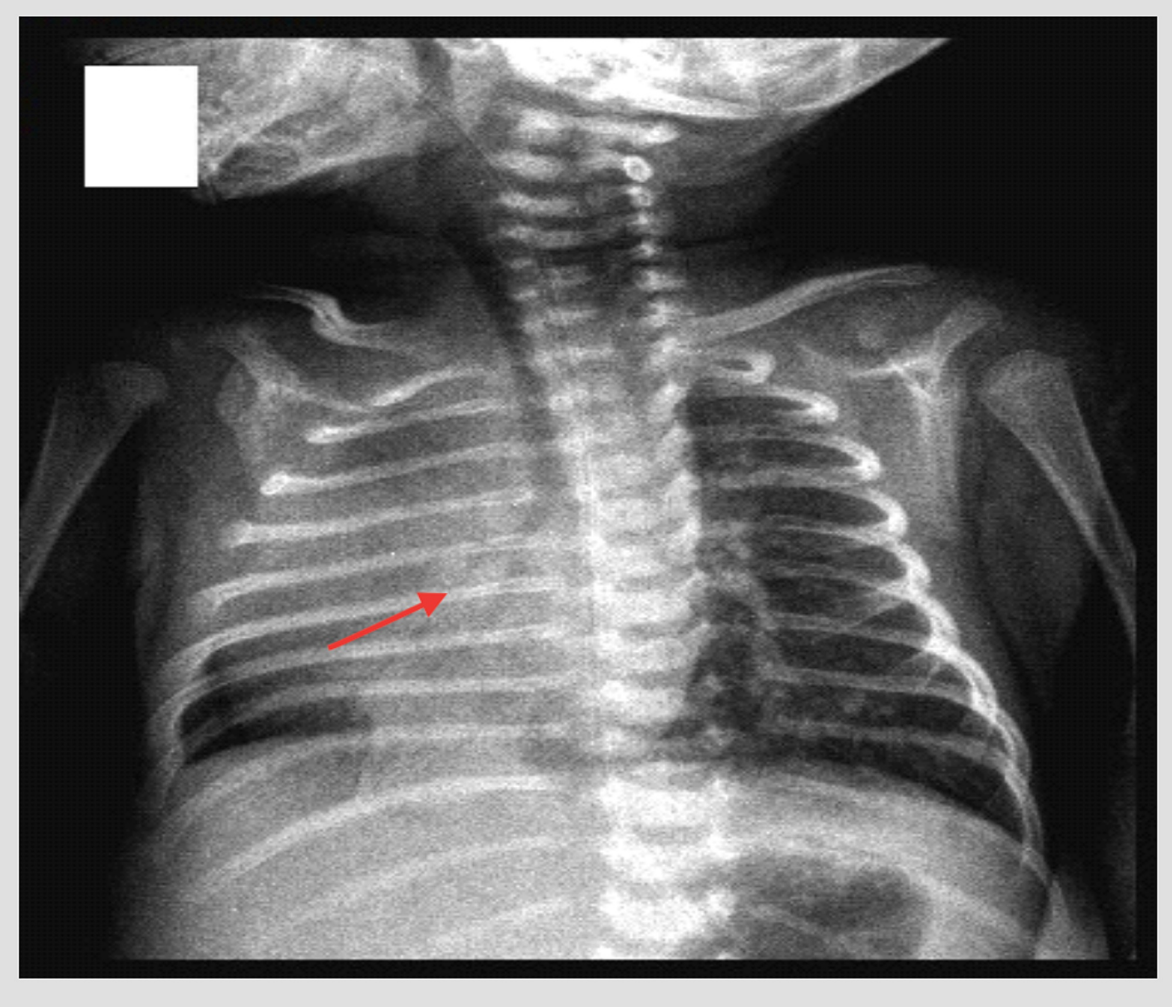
Chest x-ray of a 15 days old boy with Scimitar syndrome shows dextroposition of the heart.
